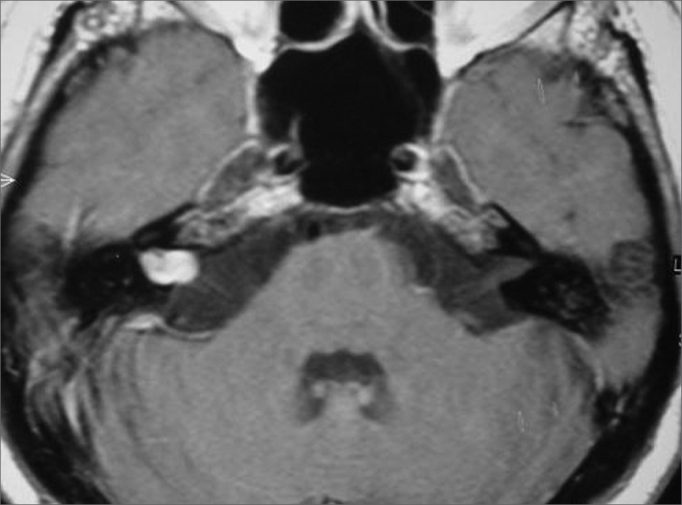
Skull MRI scan (axial T1 contrast-enhanced) showing right-side cavernous hemangioma.
